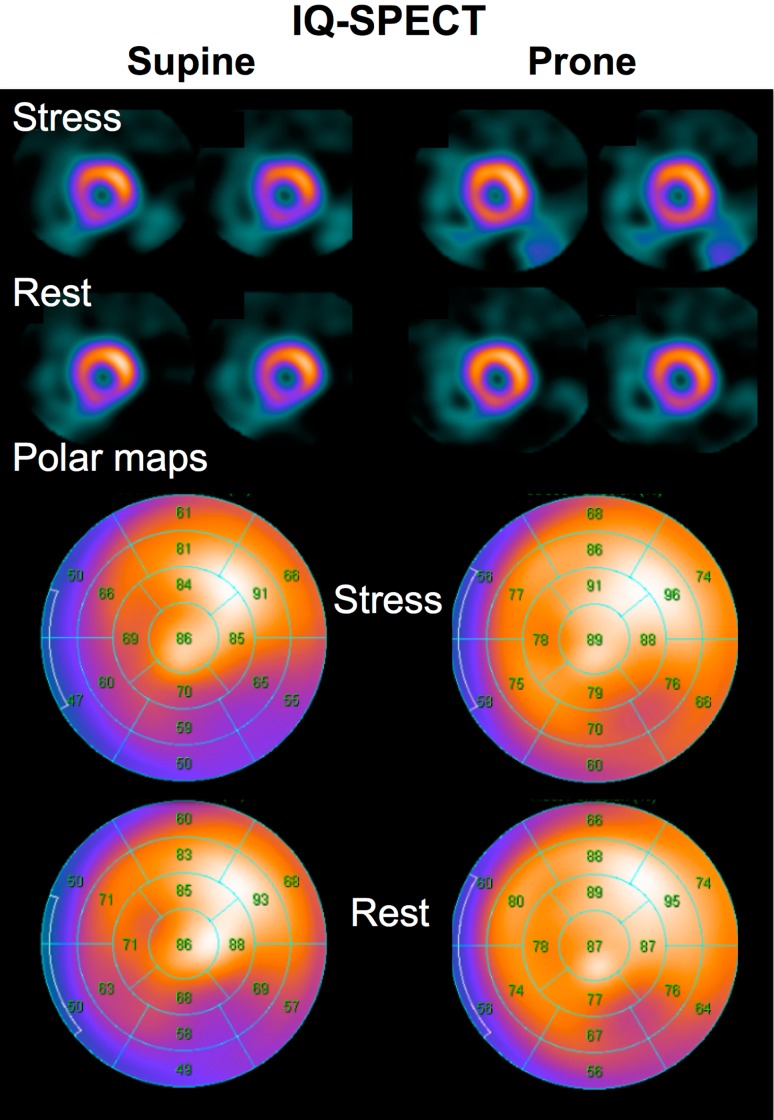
Comparison of IQ·SPECT images in the supine and prone positions. The prone image improves low counts in the inferior wall that is observed in the supine position
